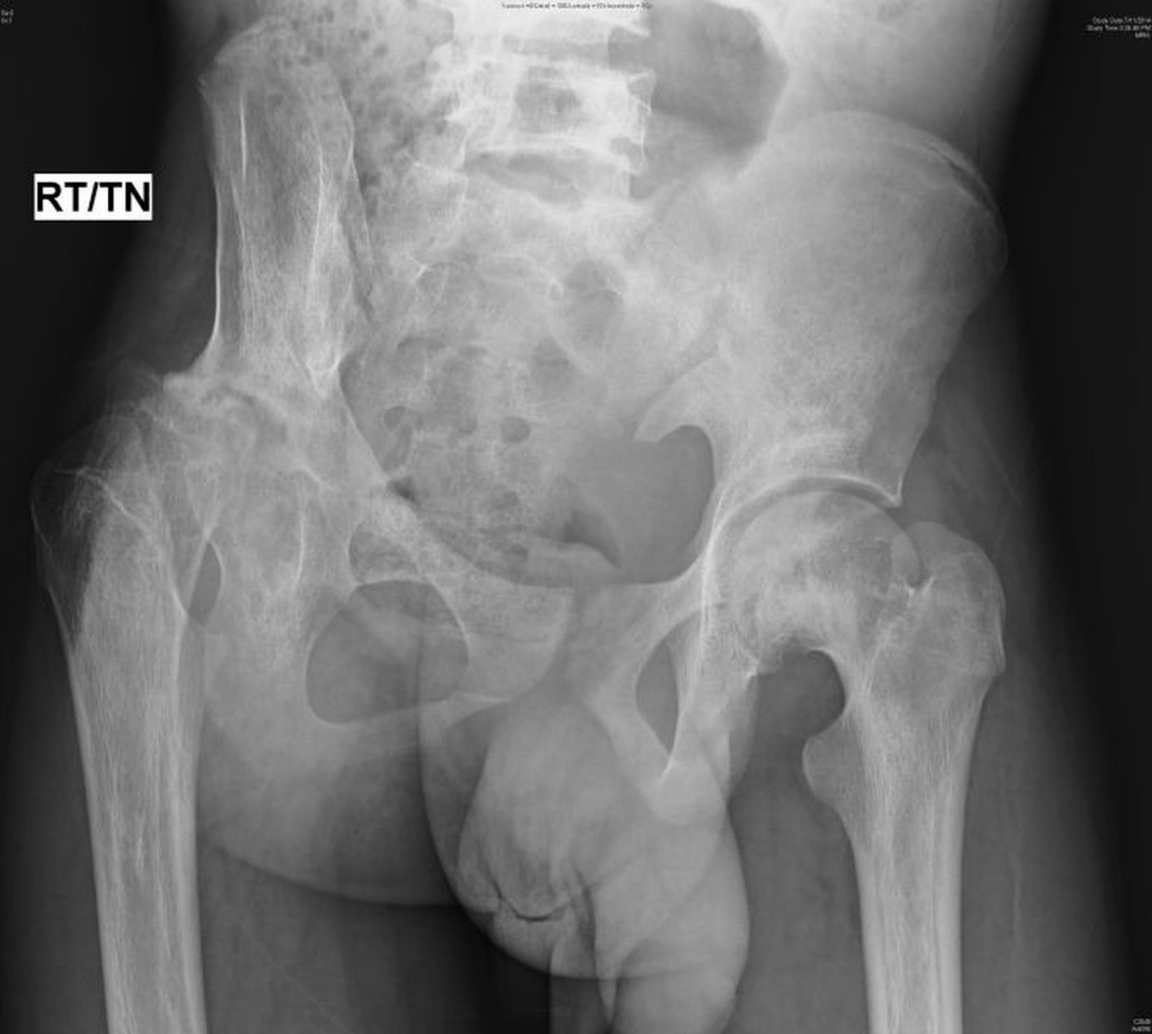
X-ray pelvis anteroposterior (AP) view showing retardation in growth of the proximal femur
